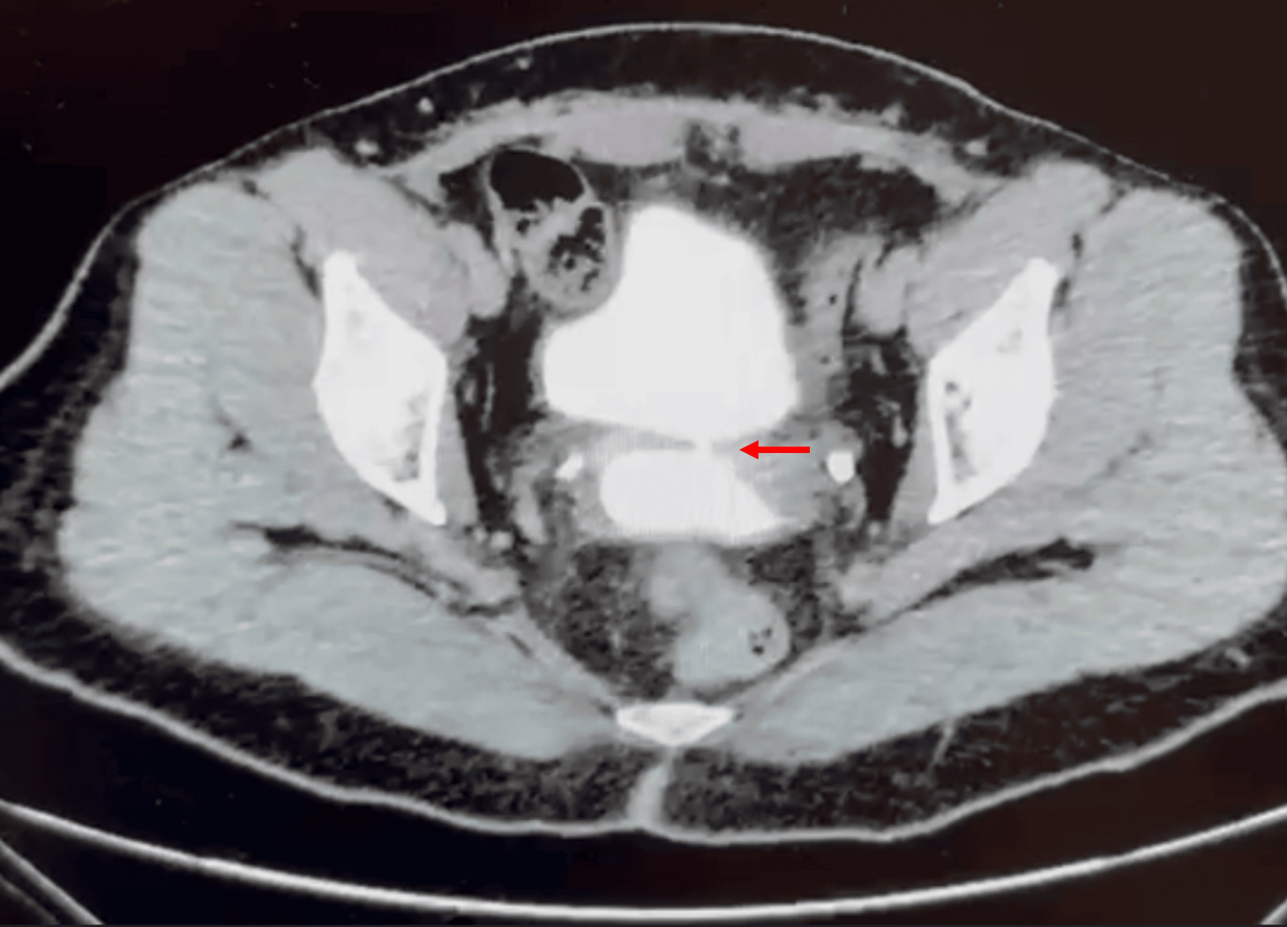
Axial delayed-phase contrast-enhanced computed tomography (CECT) showing a vesicovaginal fistula. Axial CT scan with intravesical contrast showing a 2 mm vesicovaginal fistulous tract (red arrow), with intravesical contrast passing through the fistula to the vagina of one of the patients included in the study.
